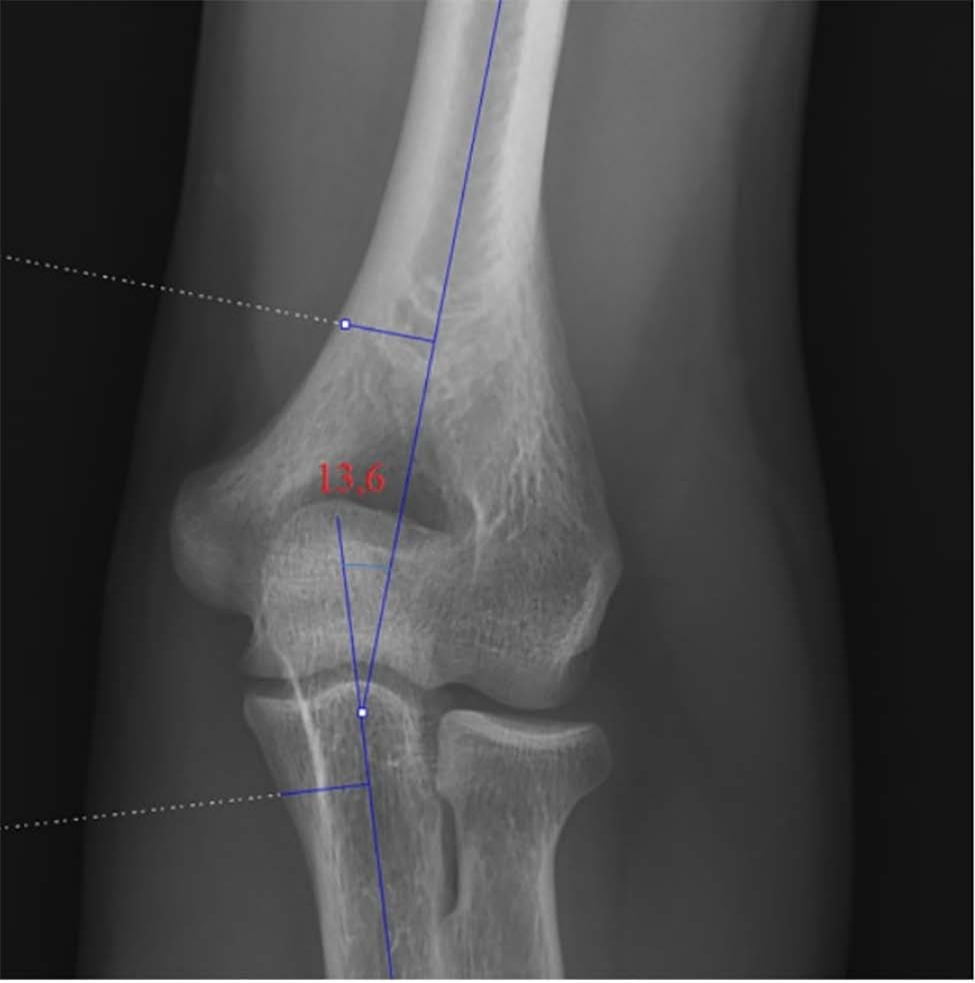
Demonstration of the measurement of elbow carrying angle on an elbow AP radiograph. AP = anteroposterior.
